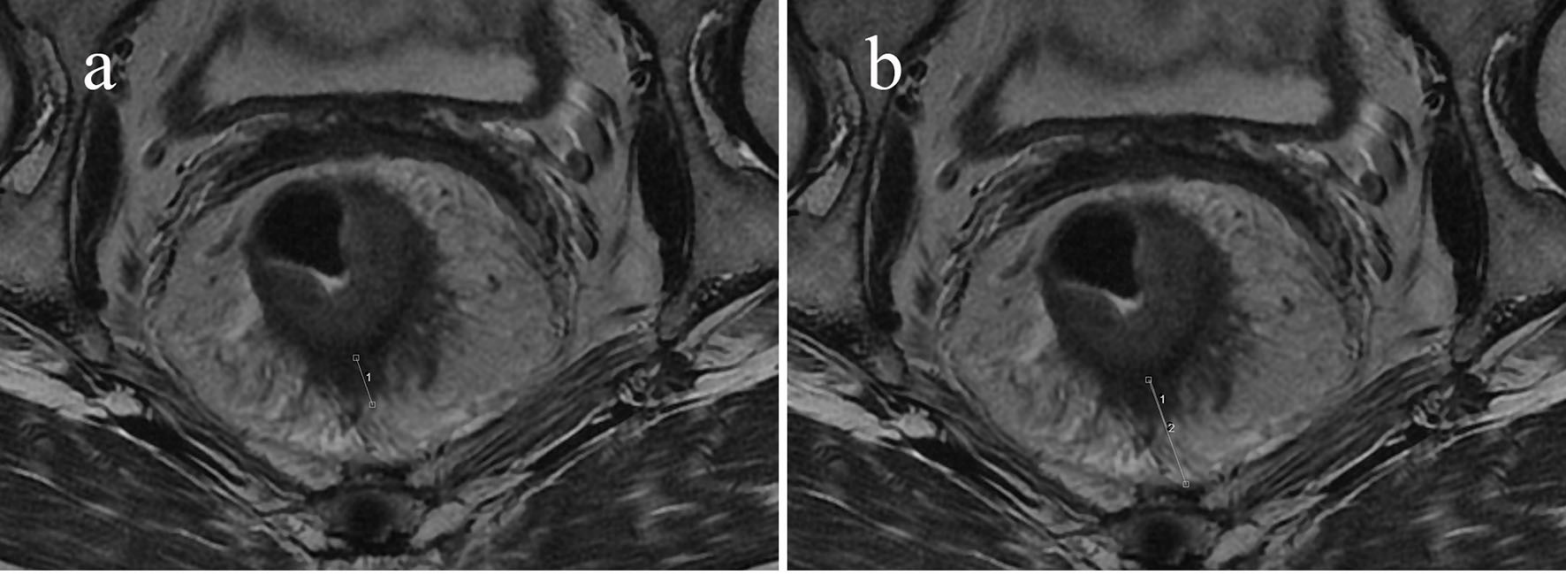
Sixty-seven-year-old man with an mr-T3N0 rectal adenocarcinoma 9.5 cm from the anal verge. Pretreatment rectal MRI (A, B) before NCRT. The EMD was 9.7 mm (A), the TM was 21.7 mm (B), and the calculated PTI was 44.7%. The rectal cancer had regressed after NCRT. The postoperative staging confirmed by pathology was T0N0, and the tumor response was pCR.
